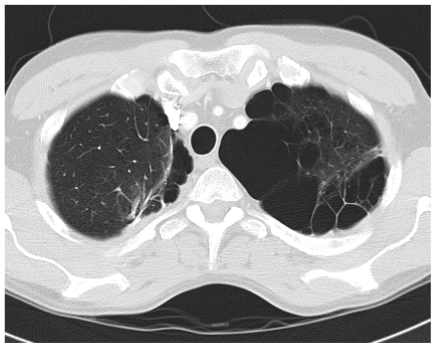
Patient 1 Postoperative CT thorax showing many large lung bullae in left apex with smaller bullae, and a bullectomy suture line in the right. The aspergilloma and surrounding cavity that was found has been excised.
